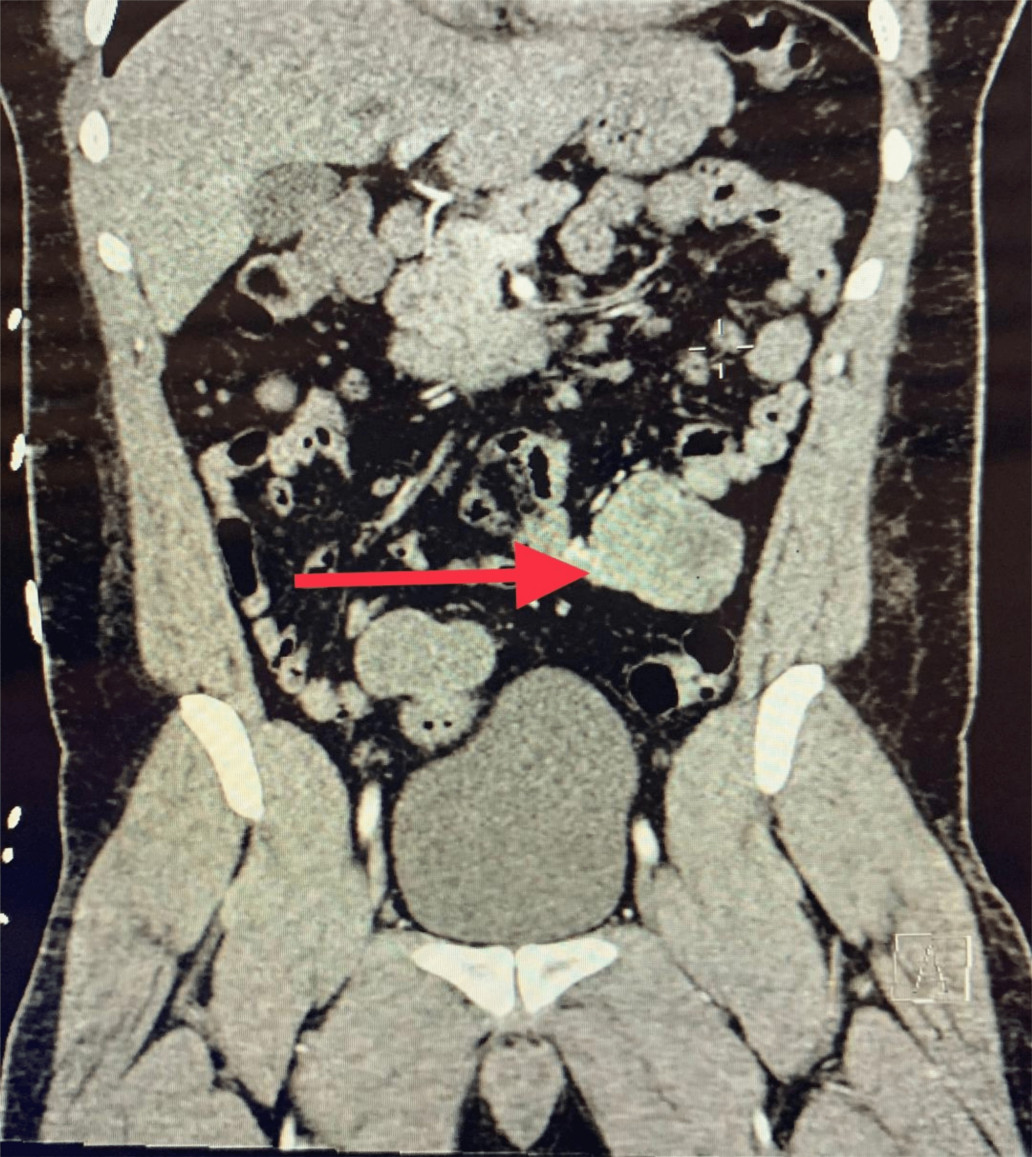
CT (coronal) of the abdomen and pelvis Soft tissue mass associated with small bowel loops in the left lower quadrant (red arrow) CT: computed tomography
